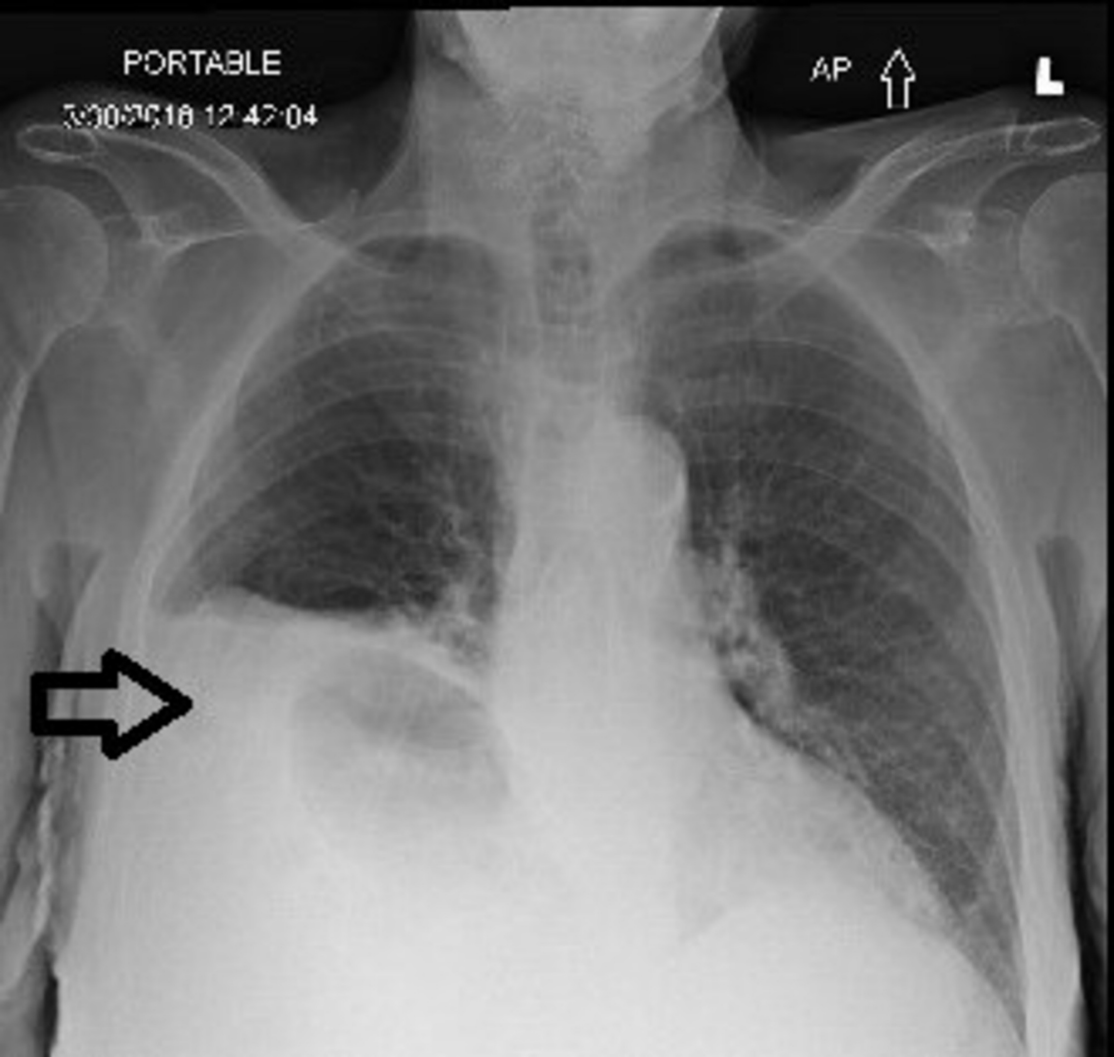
Radiograph of chest anterior-posterior view; arrow indicates the elevation of the right hemidiaphragm with diaphragmatic hernia
